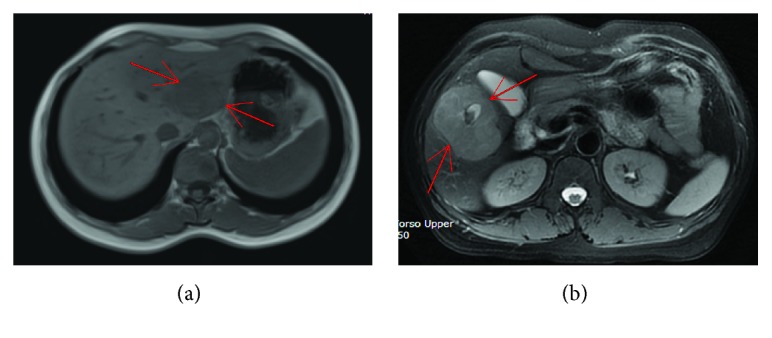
Image examination of primary hepatic neuroendocrine carcinomas. (a) MRI revealed the size of about 5.5 × 4.0 cm, slightly longer T1 and T2 signal masses, a clear boundary, and an uneven internal signal in the left lateral lobe of the liver. (b) Contrast-enhanced MRI revealed an irregular mixed appearance in the right lobe of the liver.
